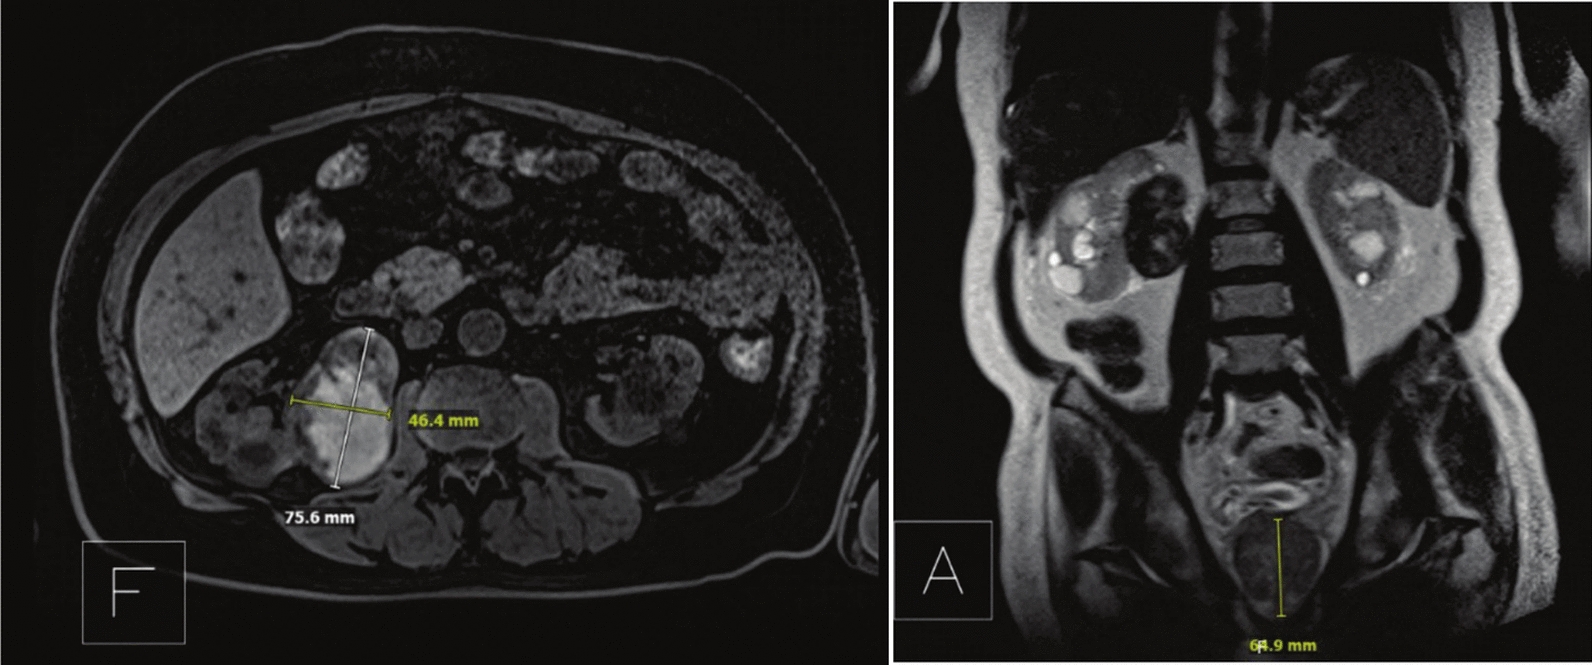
Case 2: Axial and coronal MRI slices demonstrated a large right renal mass and enlarged prostate
